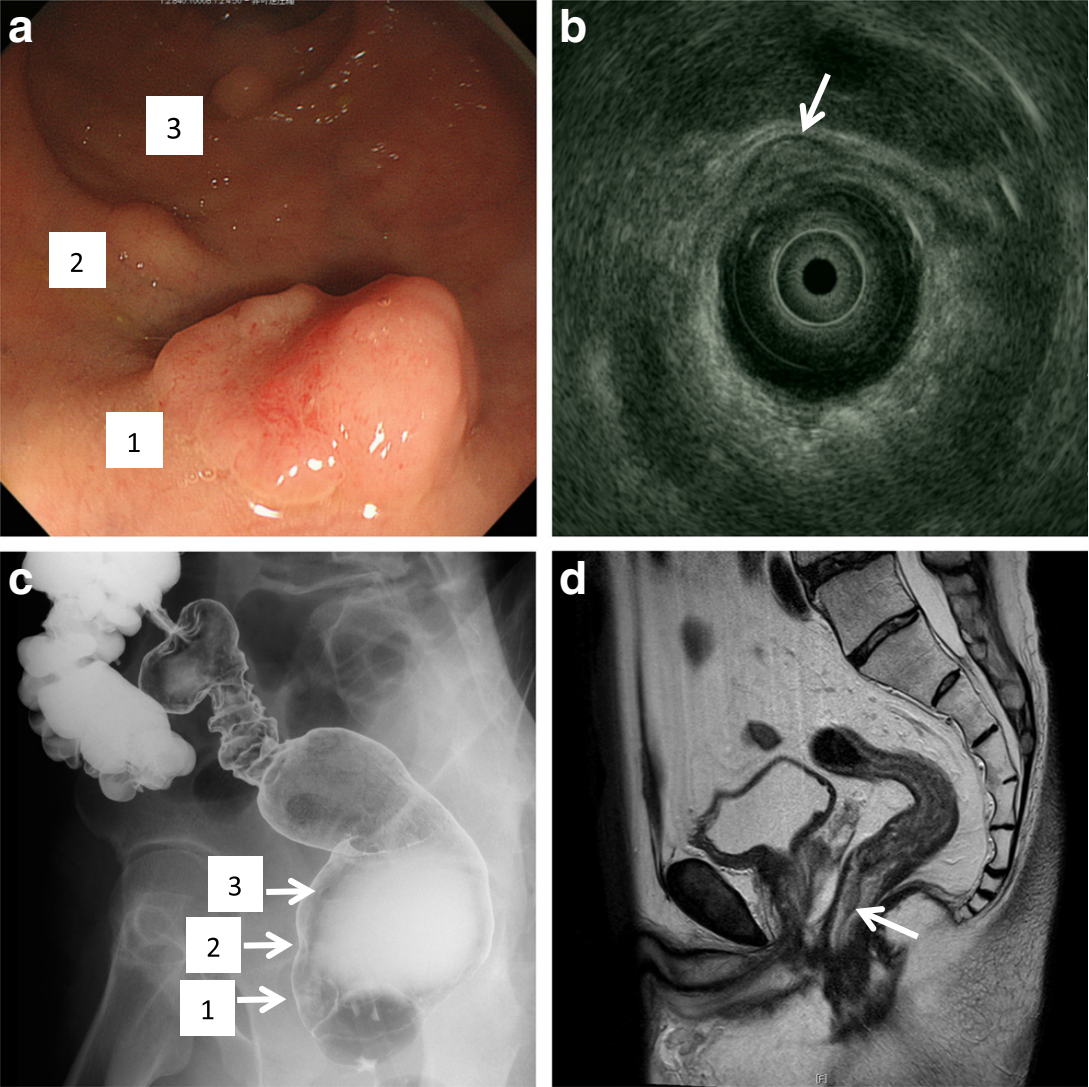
a Colonoscopy revealed a primary rectal cancer (*1*), a submucosal tumor (*2*), and an adenomatous polyp (*3*). b Transrectal ultrasonography showed that the invasion depth of rectal cancer was the deep layer of the submucosa but not the muscularis propria. c Barium enema examination showed that a rectal cancer with an irregular surface (*1*), a submucosal tumor with a smooth surface (*2*), and an adenomatous polyp (*3*) are located at the anterior wall of the rectum. d MRI showed that the rectal cancer was located at the anterior wall of the rectum without invasion into the prostate
